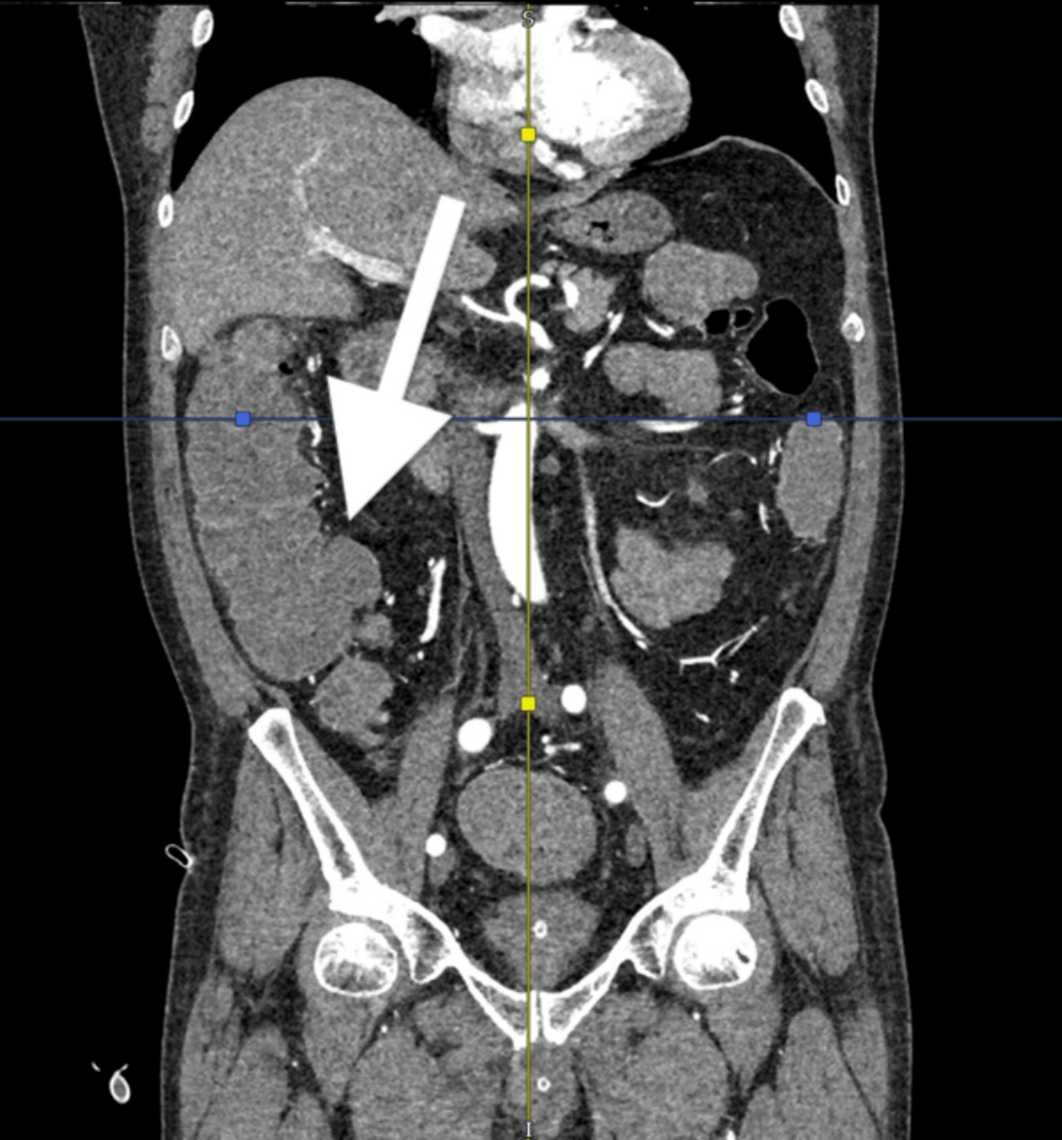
Portal venous phase coronal section of a CT angiogram demonstrating diverticulosis of the ascending colon but no contrast extravasation
